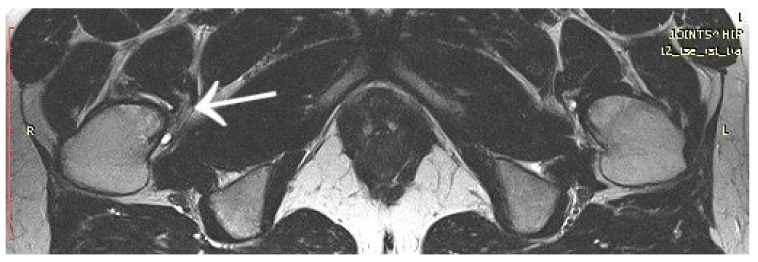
MRI scan of the hip joints; subtle changes (arrow) in the right femoral neck made suspicion of fatigue fracture, no focus of OO revealed initially.
